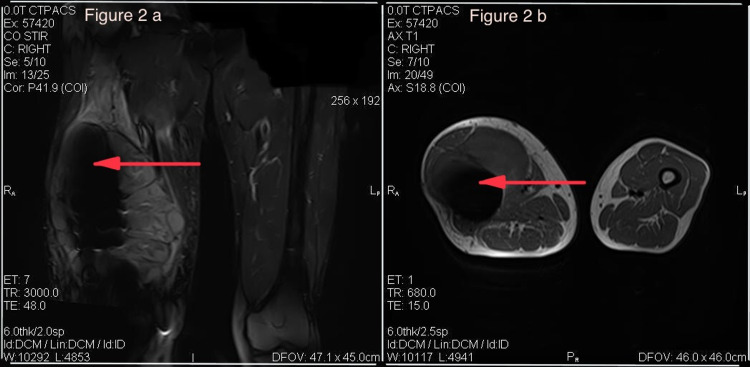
MRI right upper thigh coronal (a) and axial (b) sections showing heterogenous collection wrapping around the bone displacing the vasti muscles and mild edema signal in the adductor magnus, brevis, and longus muscles.
